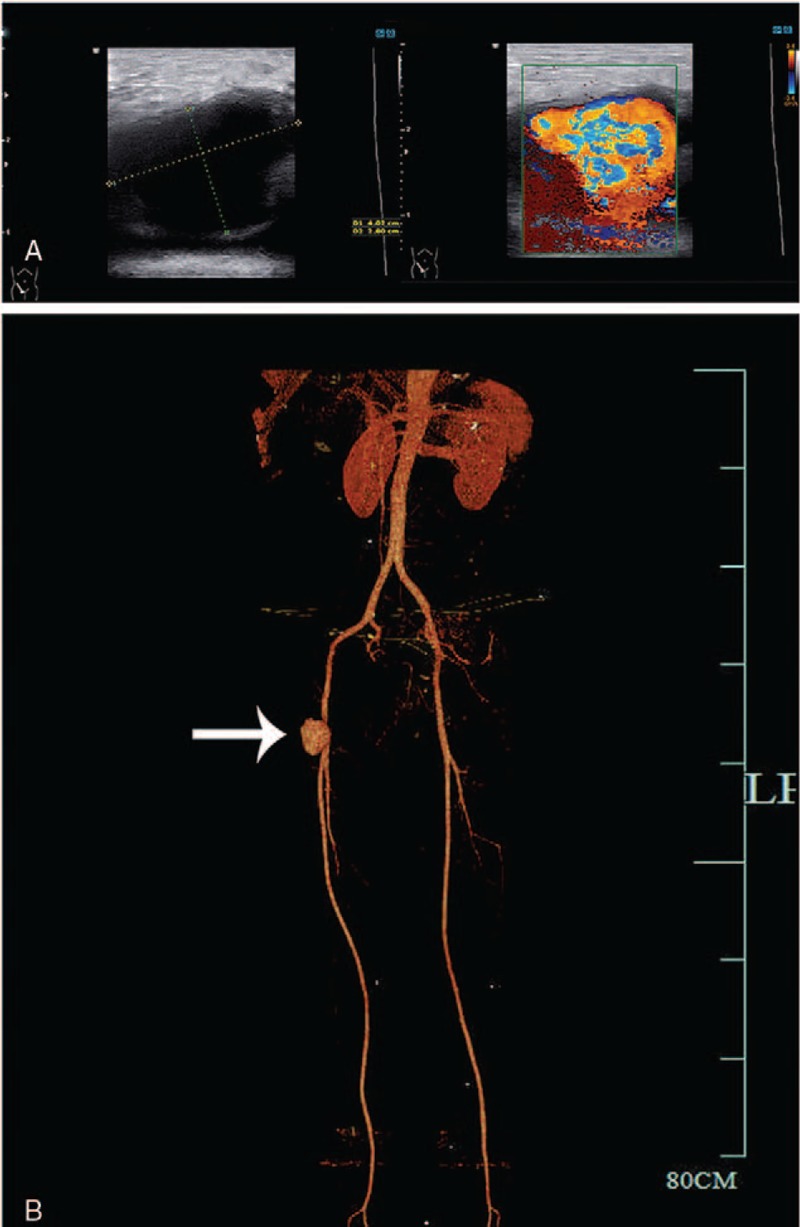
Ultrasonography and CTA in lower extremity arteries. (A) A pseudoaneurysm approximately 4 × 3 cm was confirmed by ultrasonography. (B) A remarkable pseudoaneurysm was also suggested by CTA in lower extremity arteries (arrow). CTA = computed tomography angiography.
